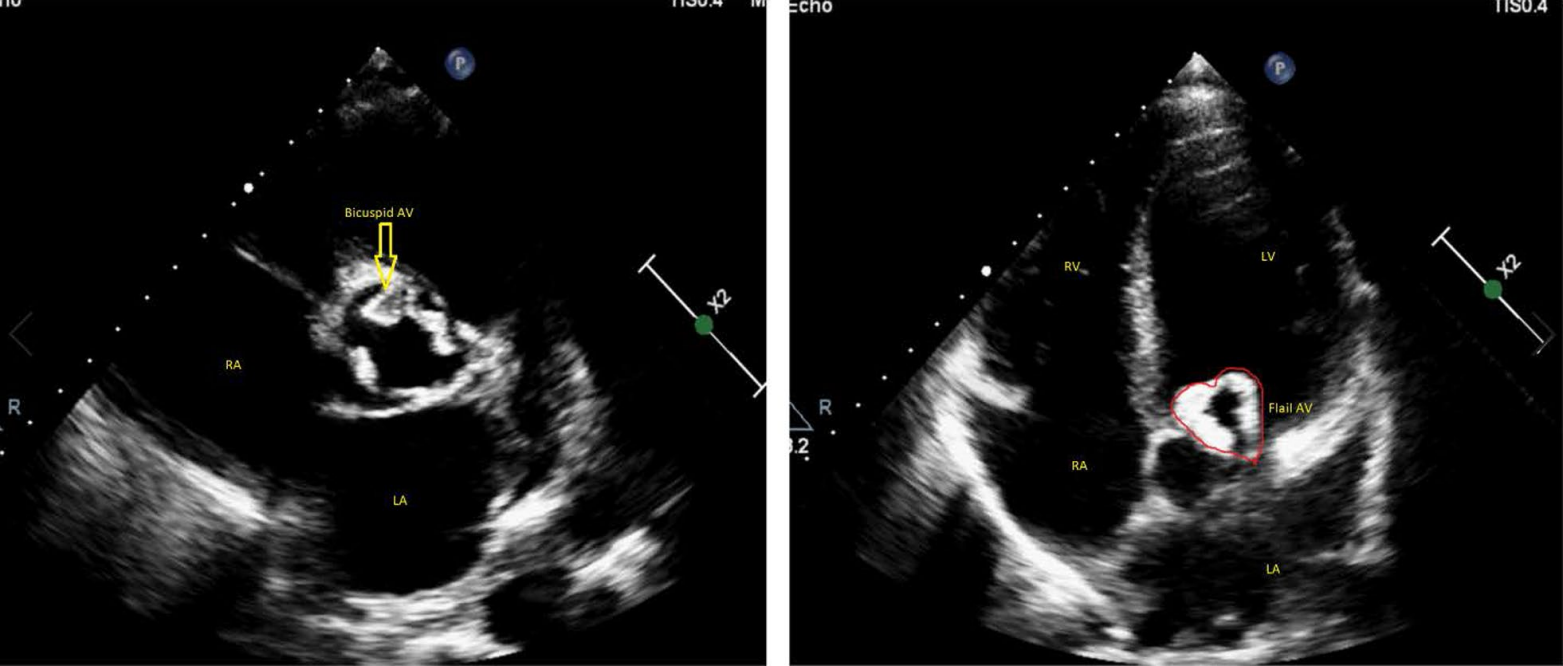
Left panel: Parasternal short axis view demonstrated thickened, calcified, and bicuspid aortic valve (arrow). Right panel: Apical five-chamber view showed flail of AV that mimics a heart configuration.
